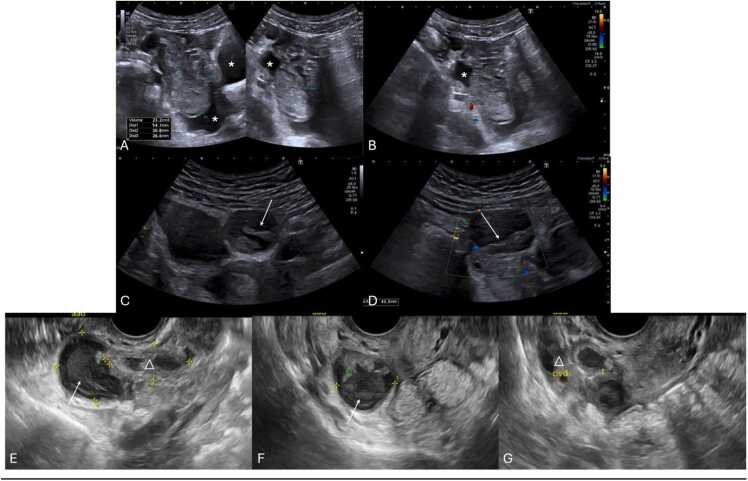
(A and B) Pelvic transabdominal b-mode and color Doppler US images in a 12-year-old female with acute onset of pelvic pain, suspected of ovarian torsion. US revealed a large, voluminous (23 cc) complex adnexal mass (between calipers in A), with no vascular flow, with associated free fluid in de the pelvic cavity (* in A and B). Due to clinical and radiological findings consistent with ovarian torsion, she was submitted to exploratory laparoscopy, which revealed the presence of a ruptured hemorrhagic ovarian cyst with no signs of ovarian torsion. (C and D) Pelvic transabdominal sagittal and transverse b-mode and color Doppler US in a 14-year-old female with acute onset of pelvic pain. US reveals a complex cystic mass with no vascular flow in the ovary, as well as the presence of the retractable cloth sign (arrow in C and D), characteristic of a hemorrhagic cyst. (E–G) Transvaginal pelvic ultrasound in an adolescent female with pelvic pain and associated fever and leukocytosis. US revealed a complex mass (arrow in E and F) adjacent to the right ovary (triangle in E and G) as well as dilated fallopian tube (t in G), allowing the diagnosis of a tubo-ovarian abscess to be made.
